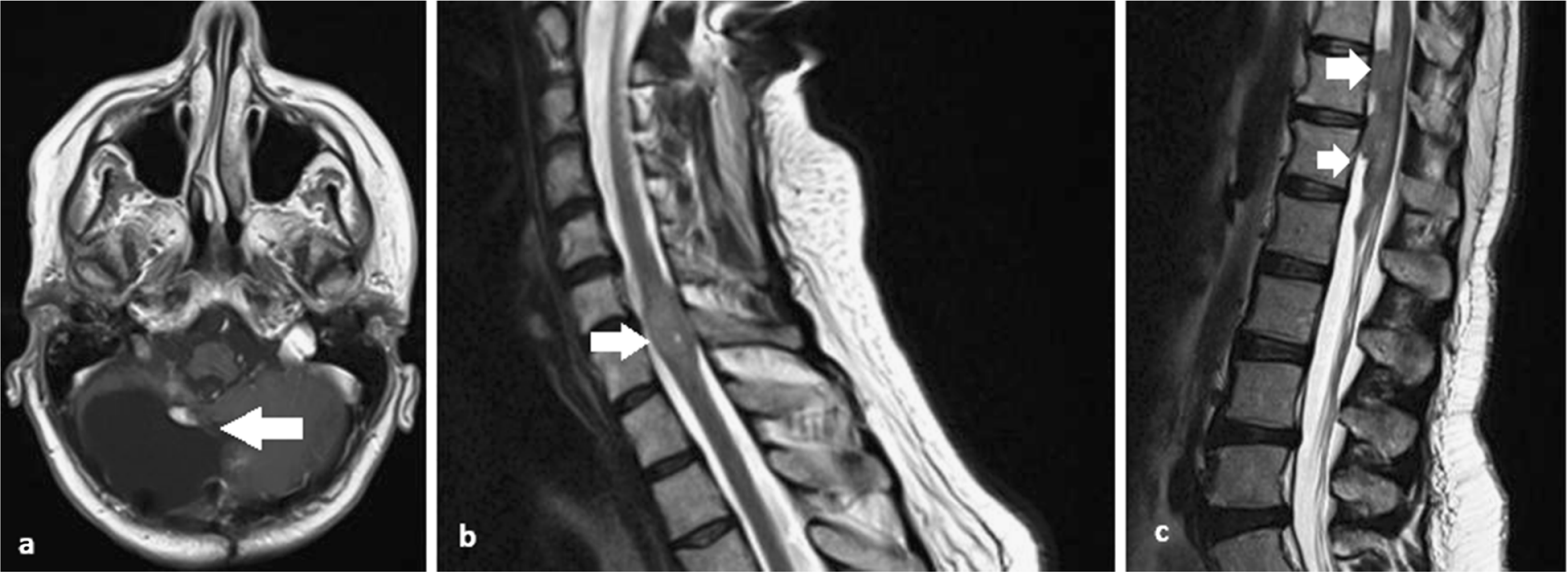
a, b and c: The magnetic resonance imaging performed at one year after re-irradiation showed disease recurrence in the spine and no brain toxicity (*white arrows*).
